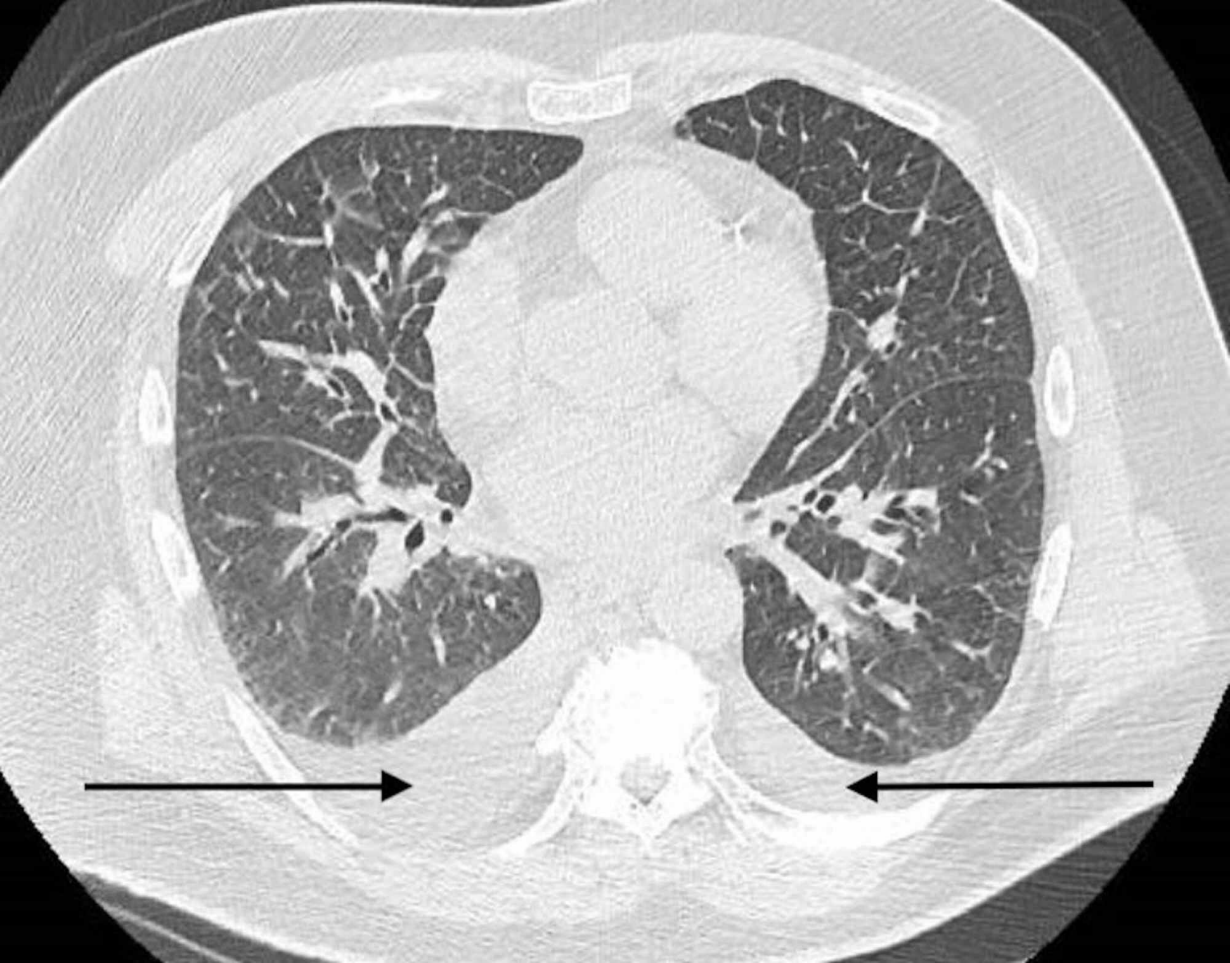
CT chest showing bilateral pleural effusions (black arrows) and pulmonary edema.
